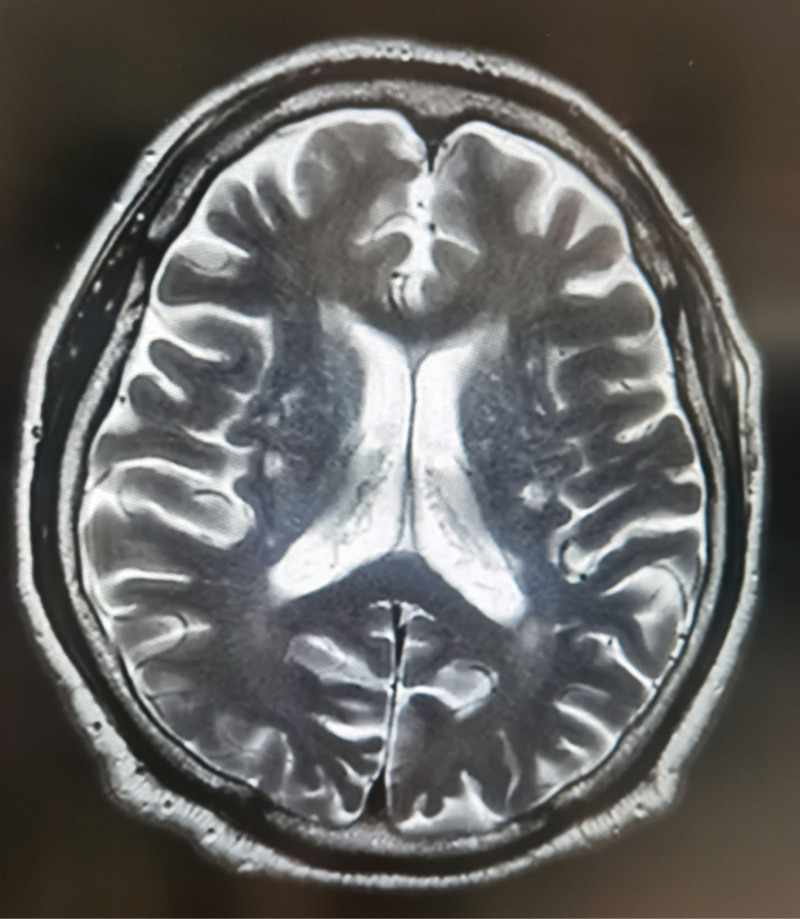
The image of brain magnetic resonance imaging (MRI). The patient showed multiple lacunar infarcts and brain atrophy, consistent with the manifestations of cerebral arteriosclerosis, and acute cerebral infarction was excluded.
